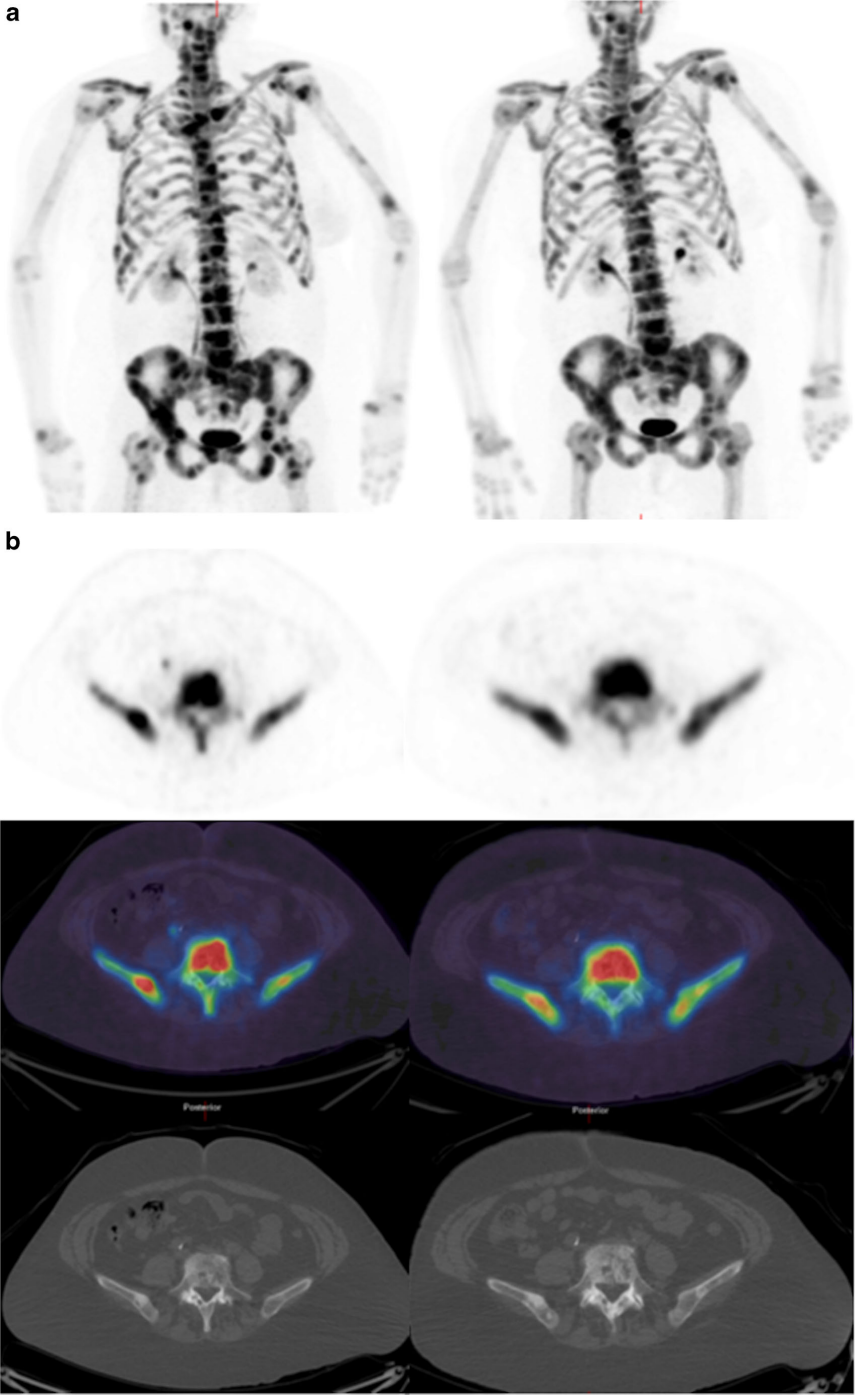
a NaF PET maximum intensity projection (MIP) and b corresponding transaxial images [PET (*top*), fused PET/CT (*middle*), and CT (*bottom*)] of a lesion in L5 at baseline (*left*) and 8 weeks (*right*) after commencing endocrine therapy in a patient who had a response by the clinical reference standard. SUV_max_ of the L5 vertebral lesion decreased from 42.7 to 32.5
